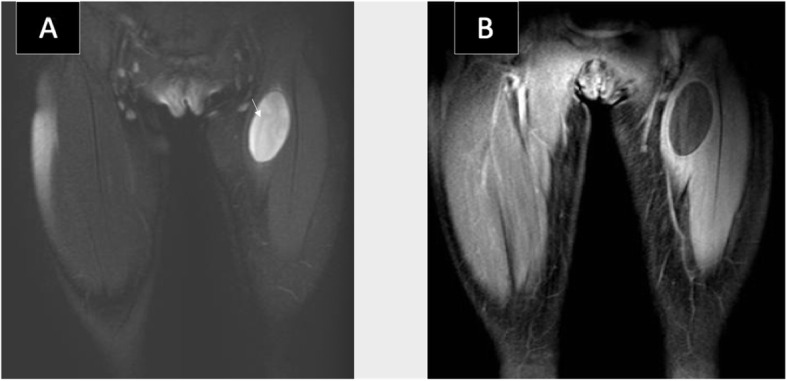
MRI of the thighs in coronal planes. a: STIR MRI image showing a well-defined cystic lesion with faint water Lilly sign (arrow) seen in the left rectus femoris muscle. b: T1 postcontrast image with fat saturation showing no perceptible enhancement of the lesion
